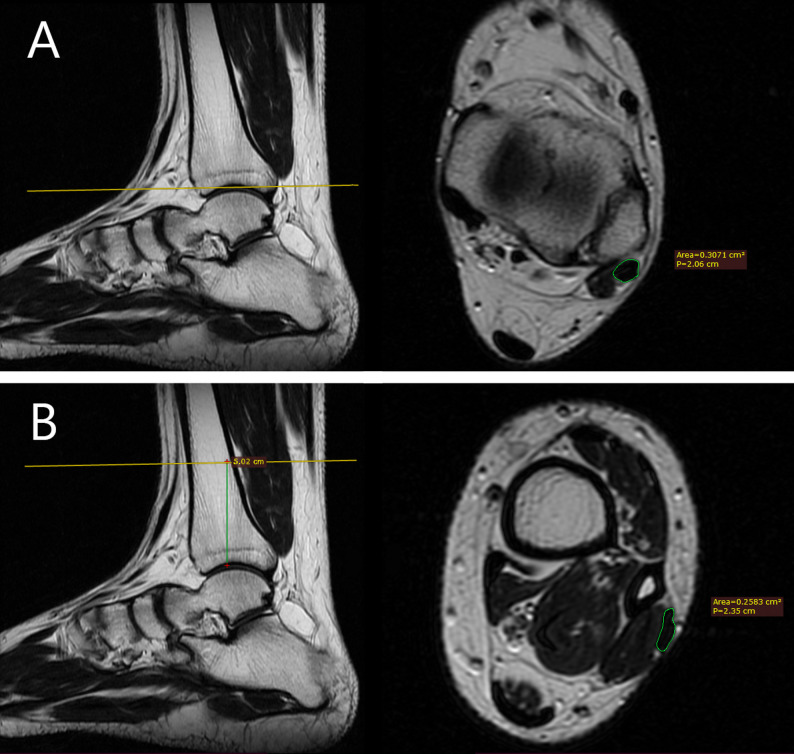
MRI images (axial image with sagittal reference for localisation) showing CSA measurement of the peroneus longus tendon at the level of the ankle joint (A) and 5 cm above the ankle joint (B)
